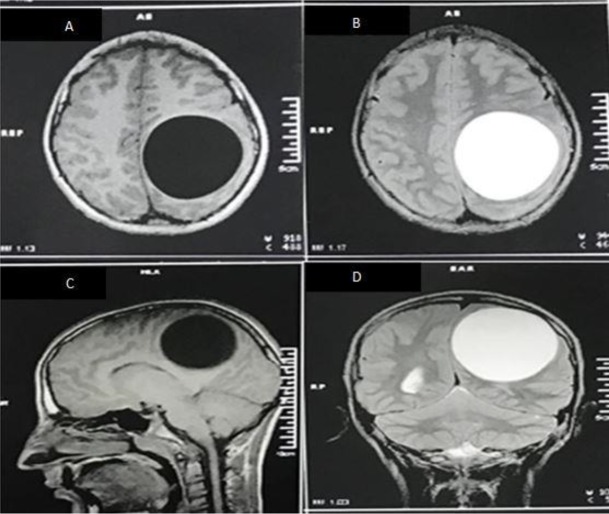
Patient’s Brain MRI A) T1W sequence in axial view, shows a hypo intense oval shaped intraparenchymal cystic lesion with distinct borders in the left parietal lobe B) T2W sequence in axial view, a hyper intense oval shaped intra-parenchymal cystic lesion C) Sagittal view of T1W sequence D) Coronal view of T2W sequence (Original images)
